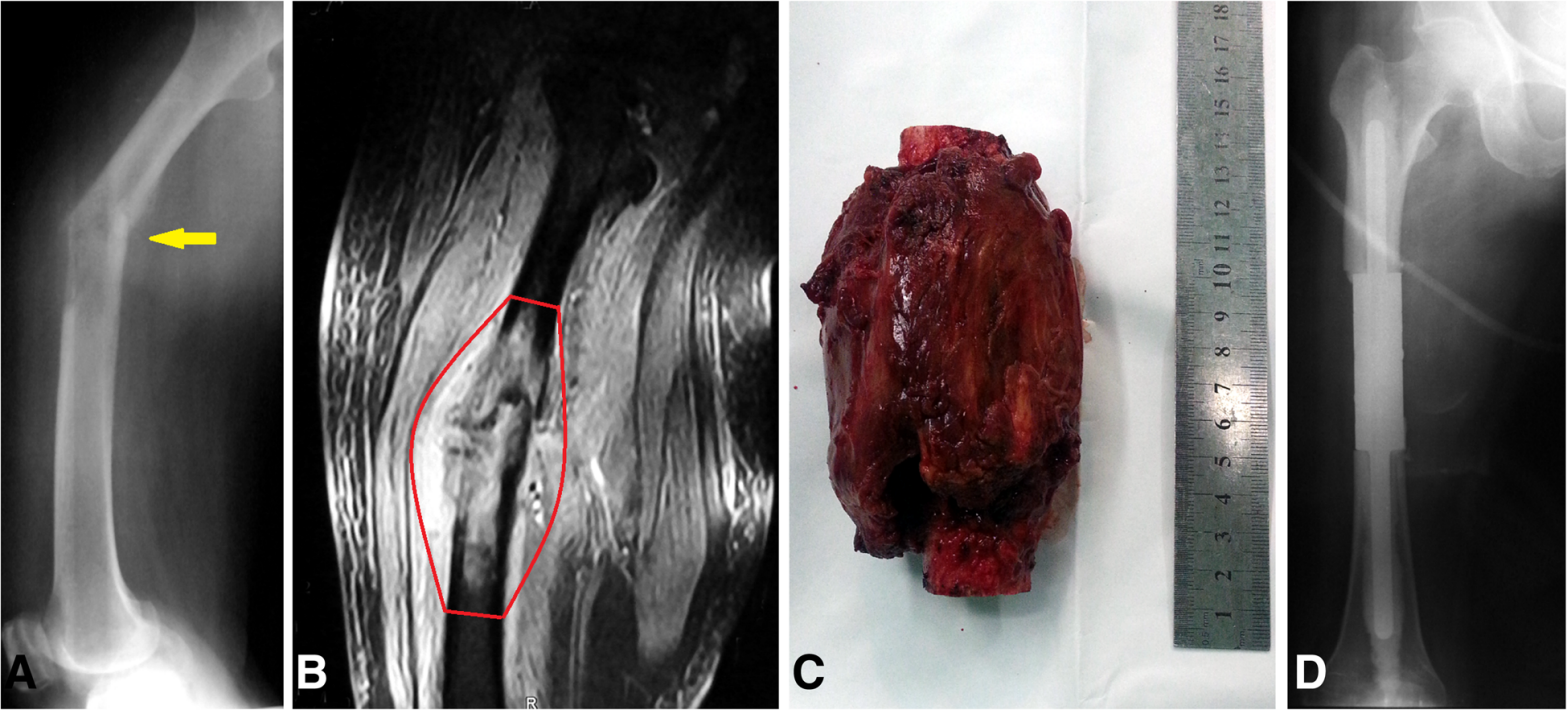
All patients underwent en bloc resection of tumors, followed by reconstruction with second-generation modular intramedullary segmental prostheses characterized by a lap joint. a Radiograph of the right femur and (B) coronal magnetic resonance imaging of the upper leg of a 74-year-old woman with rectal carcinoma involving the diaphysis of the femur (Patient 16). The yellow arrow in a showing the site of the femoral pathological fracture. The red line in b showing the surgical margin. c En-bloc resection of a malignant bone tumor. d Postoperative radiograph of the femur at 2 months after surgery, showing a stable diaphyseal construction
